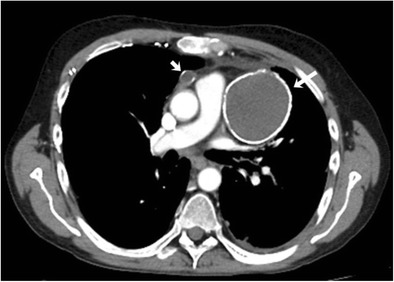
A 37-year-old male with a history of HL treated with chemotherapy and mediastinal radiation 5 years ago. Axial contrast-enhanced CT image reveals anterior mediastinal lymphadenopathy with peripheral calcification (*arrows*)
